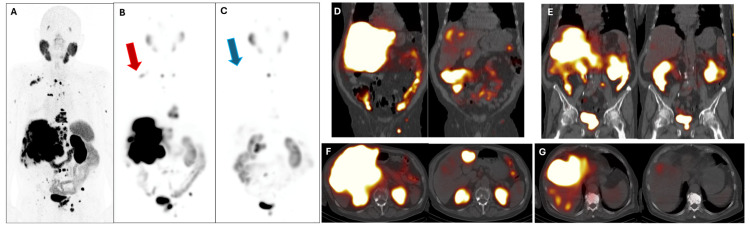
The 80-year-old patient was referred to PRLT due to mCRPC with neuroendocrine differentiation (Gleason score 9). The patient had received local therapy of the primary tumor after diagnosis (cT4, 2014), therapy with goserelin (2016–2020, resumed from 2022 due to local recurrence with rectal infiltration, bone, and LNM), enzalutamide, and denosumab. In August 2024, a rising PSA level (up to 71 ng/mL) prompted a [^68^Ga]Ga-PSMA PET/CT (A), MIP, which showed progression with local recurrence, extensive bilobar liver metastases, disseminated bone metastases, and LNM. Consequently, the patient was administered the first cycle of [^177^Lu]Lu-PSMA (9.0 GBq) combined with benfo-oxythiamine, which was well tolerated (beginning of August 2024; PSA 79.5 ng/mL). Post-therapeutic SPET/CT the day after injection (B), MIP revealed intense bilobar hepatic PSMA uptake («sink effect», reflecting intense PSMA accumulation in liver metastases) and uptake in bone metastases and LNM (e.g., red arrow indicating retroclavicular on the right). At the end of September 2024, the patient was administered the second cycle of [^177^Lu]Lu-PSMA PRLT (8.6 GBq, PSA 55.1 ng/mL); post-therapeutic SPET/CT (C), MIP demonstrated an overall extreme response with reduction of liver size (craniocaudal dimension decreased from 20.5 cm to 18.5 cm) and dramatic decrease of hepatic uptake of the radiotherapeutic compound. Most bone metastases and LNM showed a marked decrease in PSMA uptake (e.g., blue arrow indicating the absence of retroclavicular LNM), with only a few lesions visible at imaging (“molecular imaging response”). Coronal PET/CT (D,E), on the left, August 2024; on the right, September 2024, shows the extreme response of hepatic metastases and LNM. Axial PET/CT (F,G), on the left, August 2024; on the right, September 2024, confirmed the massive reduction of uptake by bilobar hepatic lesions.
